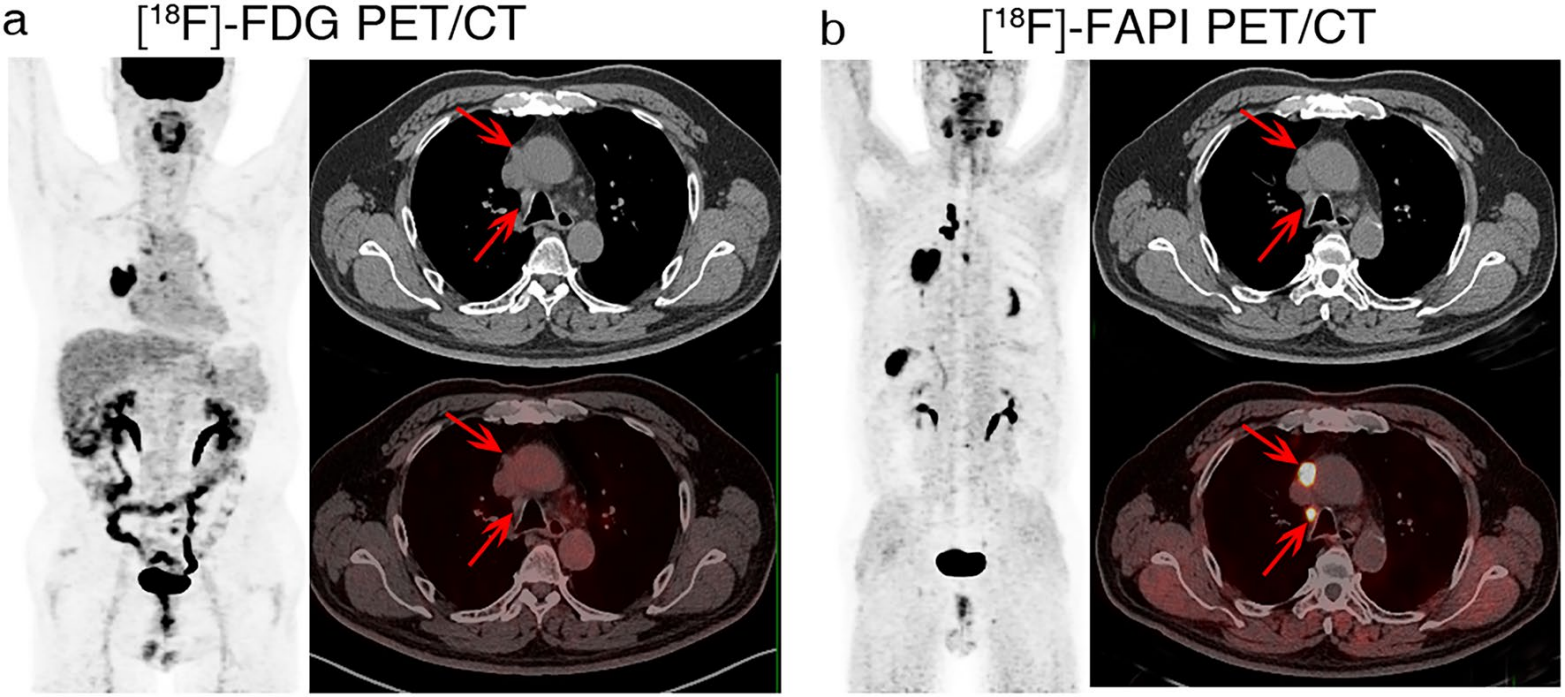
A 58-year-old man with a diagnosis of lung cancer. Small (0.7 * 0.7 cm) metastatic lymph nodes in region 4R showed no ^18^F-FDG-avidity (a, red arrows), but remarkable.^18^F-FAPI-avidity (b, red arrows)
